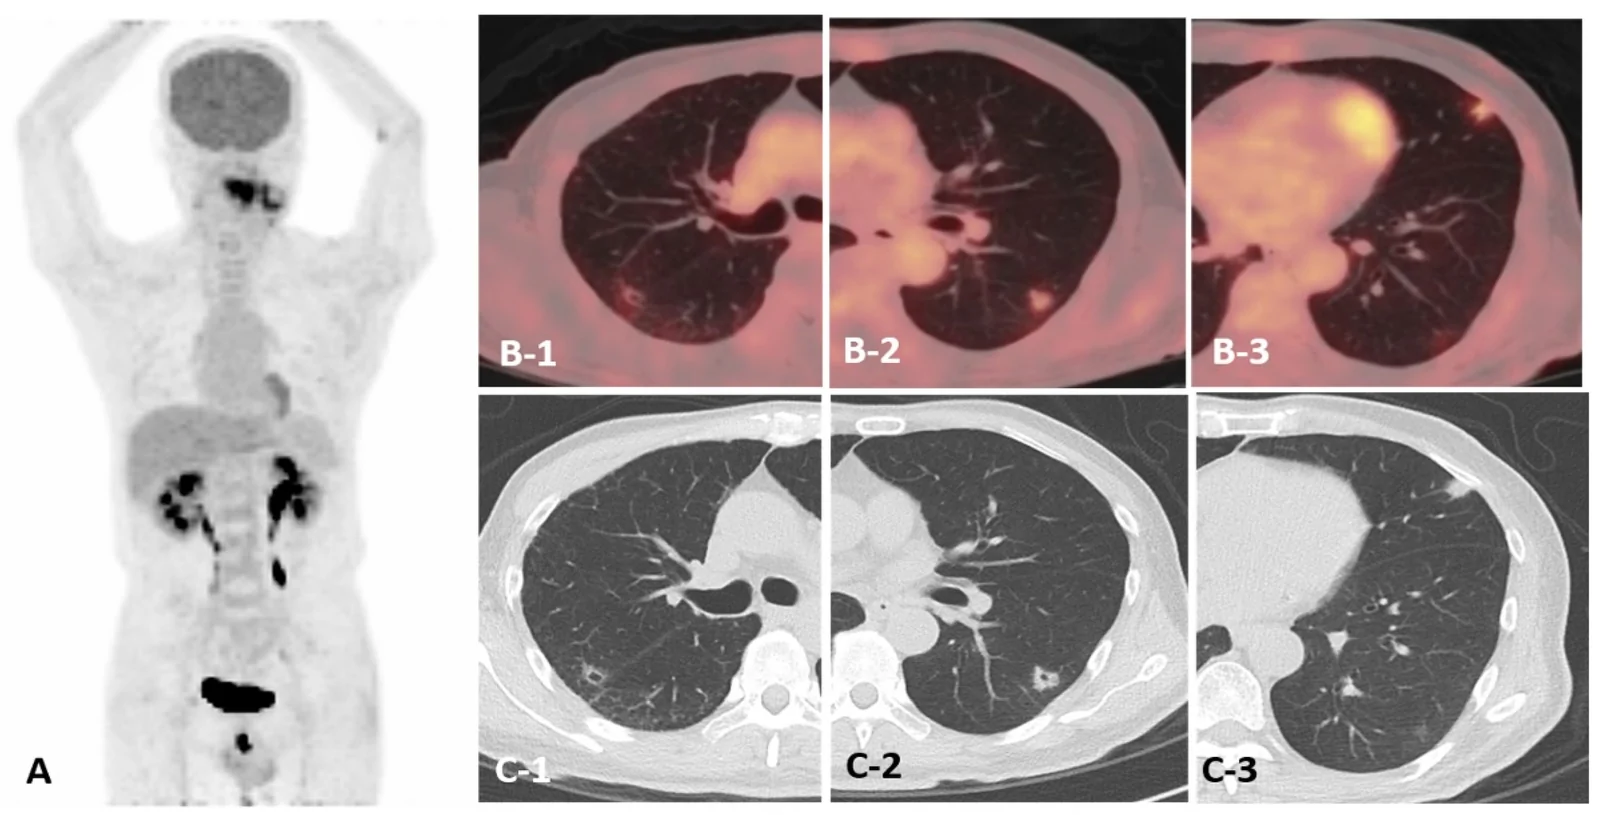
Cavitary/subsolid metastatic nodules mimicking infection. Atypical pulmonary metastases in a man with tongue cancer resemble septic emboli or fungal infection. The FDG PET/CT maximum-intensity projection (A), fusion images (B-1–B-3), and CT images (C-1–C-3) reveal multiple cavitary, irregular, or subsolid nodules with mild FDG uptake. Wedge resection of the pulmonary nodules confirmed metastatic squamous cell carcinoma.
